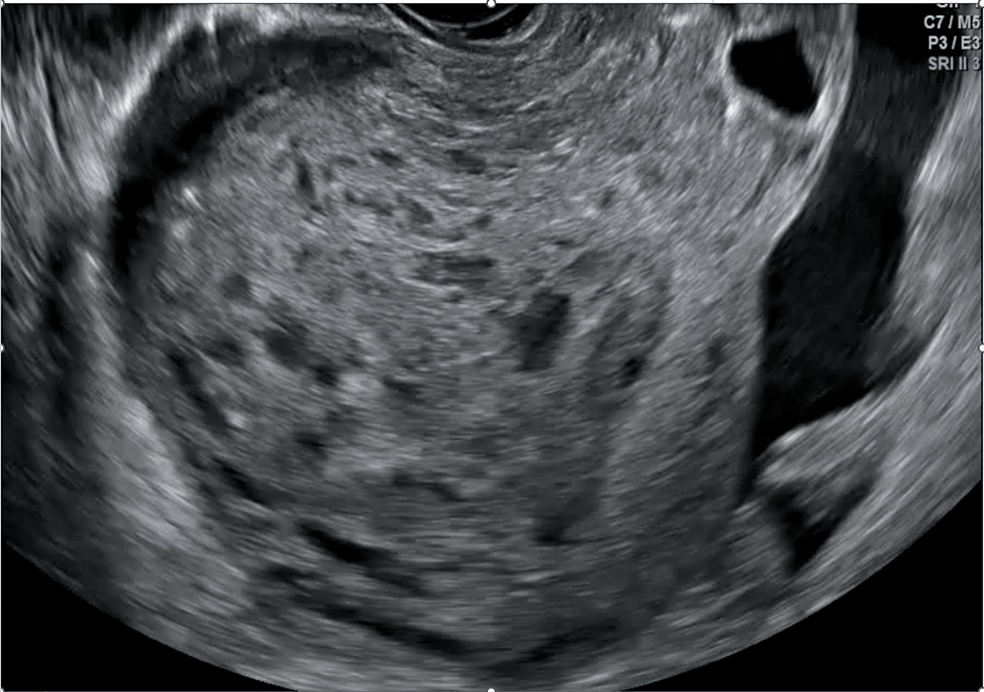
Transvaginal ultrasonography demonstrating an enlarged uterus with solid and cystic components within the endometrial cavity that penetrate the myometrium, indicating the presence of choriocarcinoma
